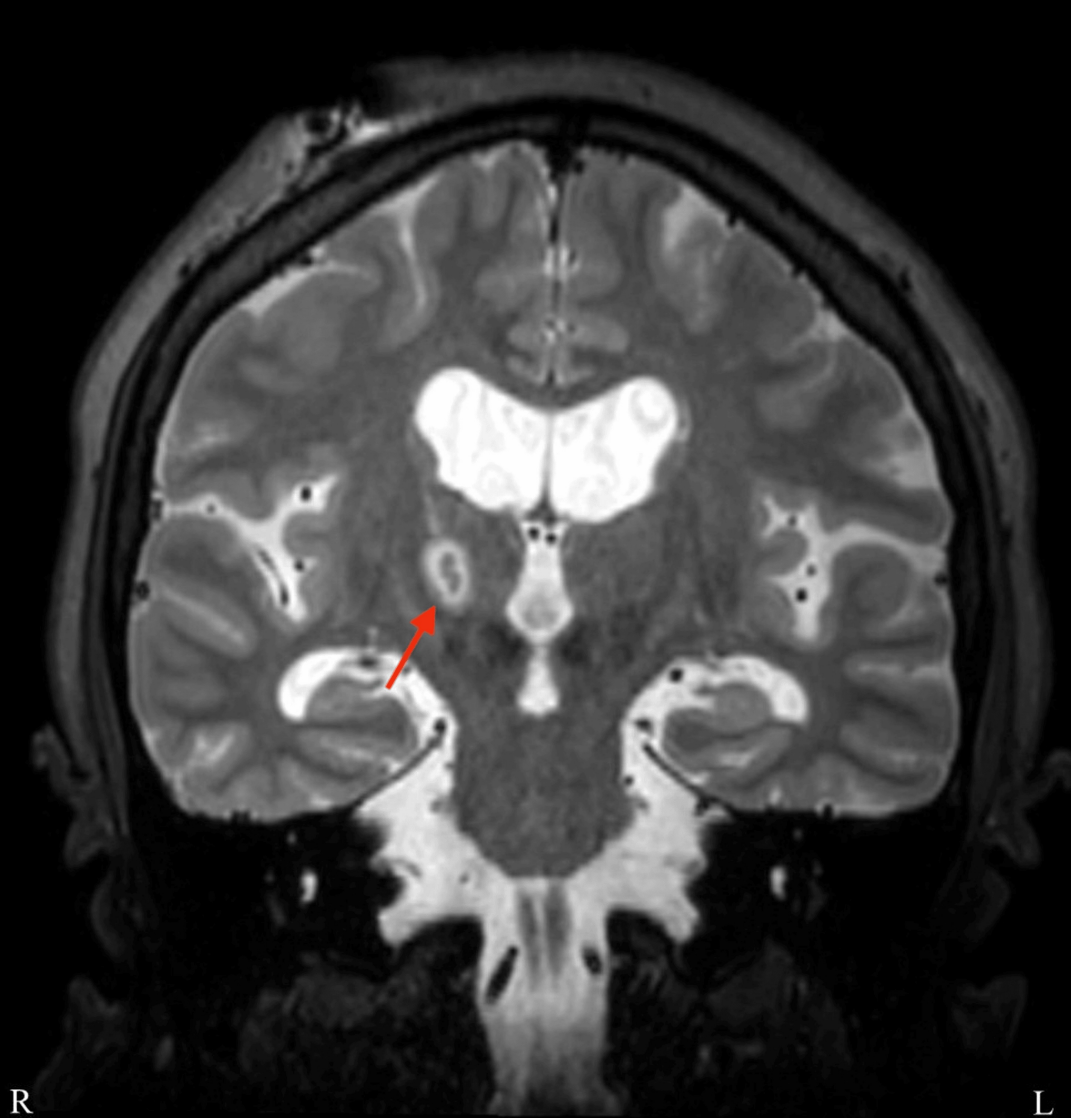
Postoperative coronal T2 weight image. As in a normal MRI, the left side of the screen is the patient's right. The coagulation area is almost identical to the previous target. The red arrow points to the lesioning area.
